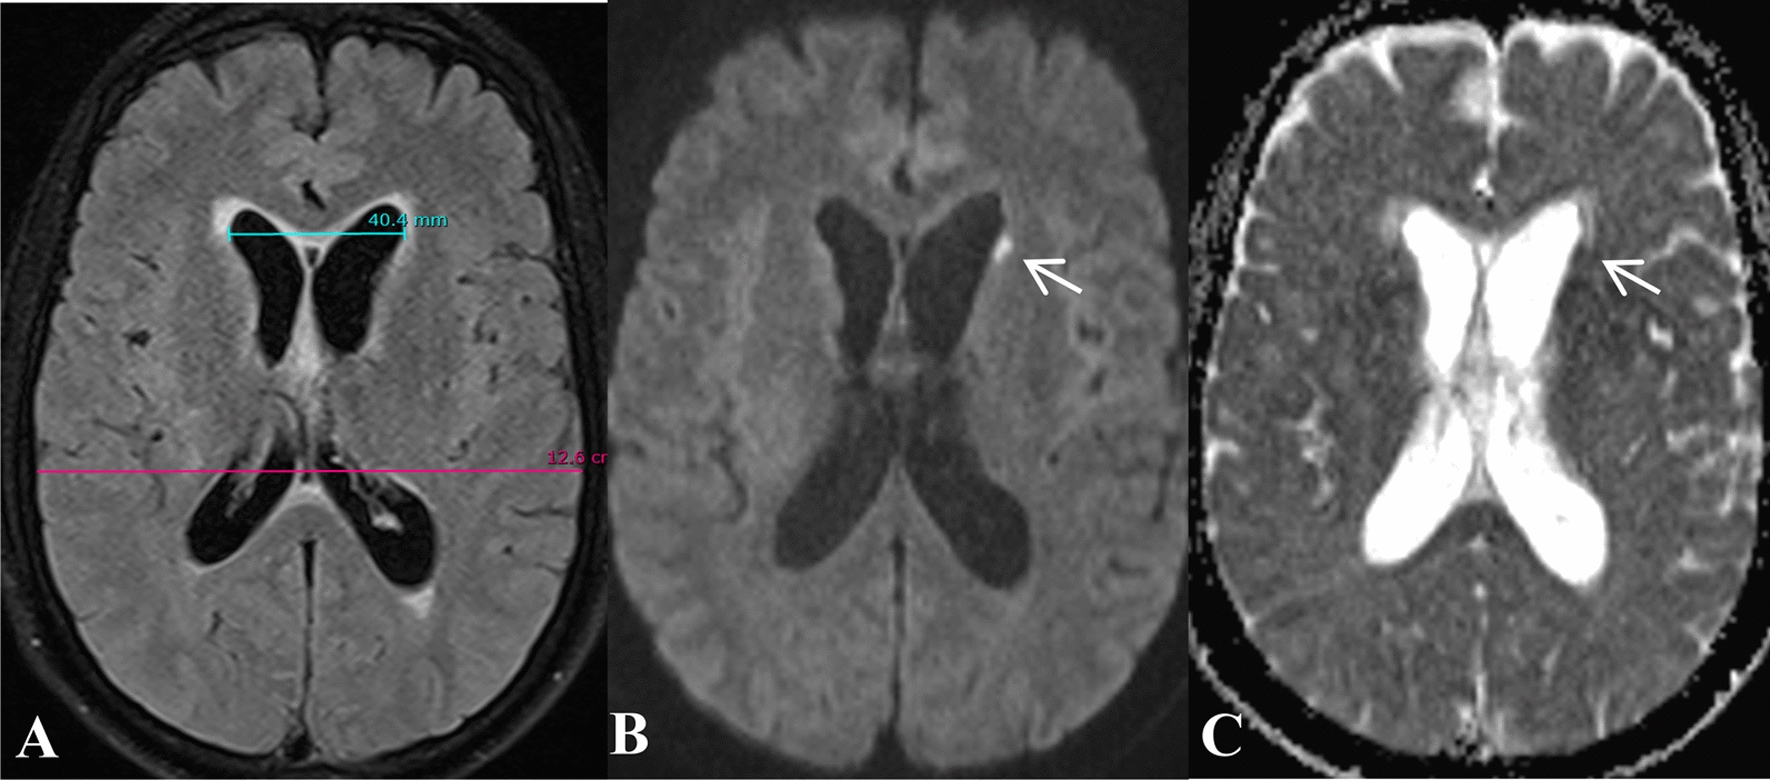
Hydrocephalus and lacunar infarct on magnetic resonance image on day 12 (re-analysis). Axial fluid-attenuated inversion recovery image (A) shows hydrocephalus with dilated frontal horns of lateral ventricles, Evans index 0.32. Axial Diffusion-weighted image (B) and ADC map (C) reveals recent lacunar infarct (arrows) with restricted diffusion at the left caudate nucleus. *NTM* nontuberculous mycobacteria
